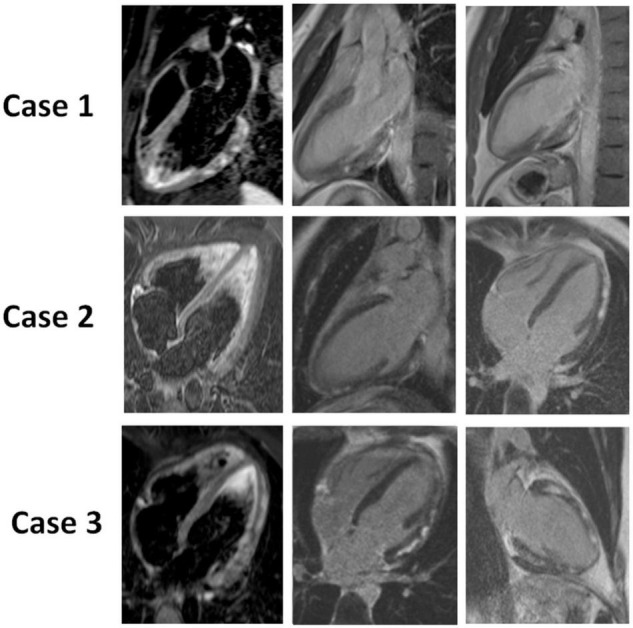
CMR of 3 patients with acute myocarditis: Case 1: a 21-year-old male patient with chest pain and ST elevation. CMR shows a marked focal edema (T2 STIR) and an intramyocardial nodular LGE of the inferolateral wall. Case 2: a 28-year-old male patient with chest pain, dyspnea, ST depression and elevated troponin. CMR shows no focal edema (T2 STIR) and a subepicardial nodular and linear LGE of the latero-apical and infero-apical walls. Case 3: a 33-year-old female patient with chest pain, palpitations, negative T waves and elevated troponin. CMR shows a moderate focal edema (T2 STIR) of the latero-apical wall and a diffuse intramyocardial and subepicardial, nodular and linear LGE. Images in the left panel of the figure are obtained in Black blood T2-weighted short-inversion-time inversion-recovery (STIR) sequence and allow the identification of edema. Images of the mid and right panel are based on inversion-recovery prepared T1-weighted sequences, performed 10 min after intravenous administration of gadolinium chelate and allow the identification of LGE.
